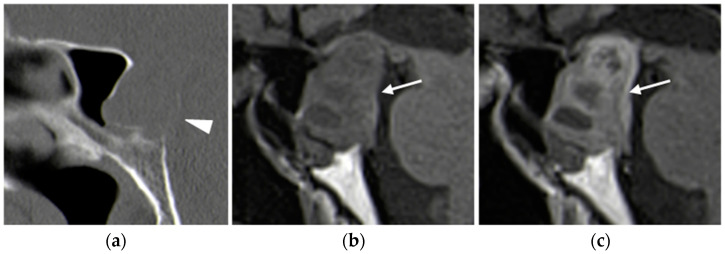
Pituitary macro-adenoma. Sagittal plane basal CT (a) image shows a large mass causing bone remodeling of the sella turcica and thinning on the posterior wall of the sphenoid sinus (arrowhead). MRI T1-weighted images before (b) and after (c) contrast administration confirm the presence of a large hypointense lesion (arrows) arising from the pituitary gland, with heterogeneous enhancement.
